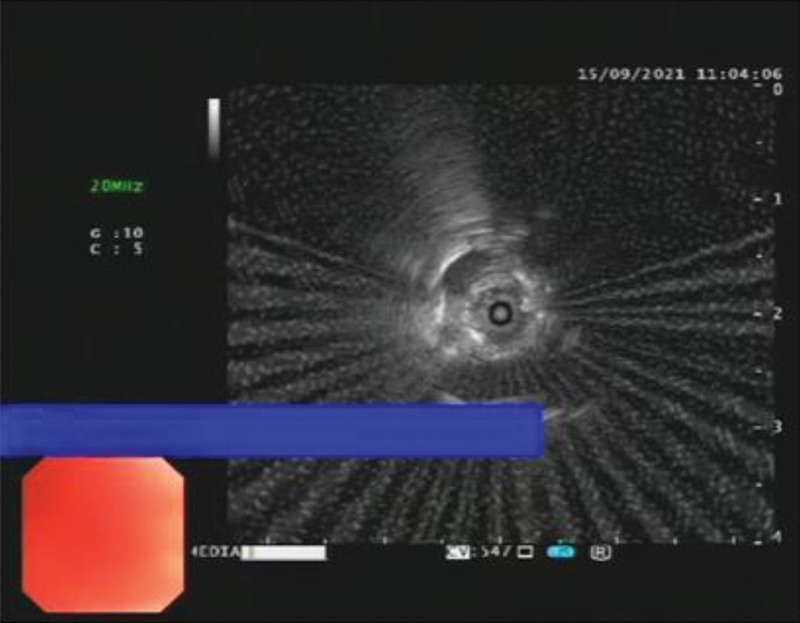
Through the tunnel formed after the puncture, endoscopic ultrasonography (EUS) was inserted again to detect abnormal echo.
